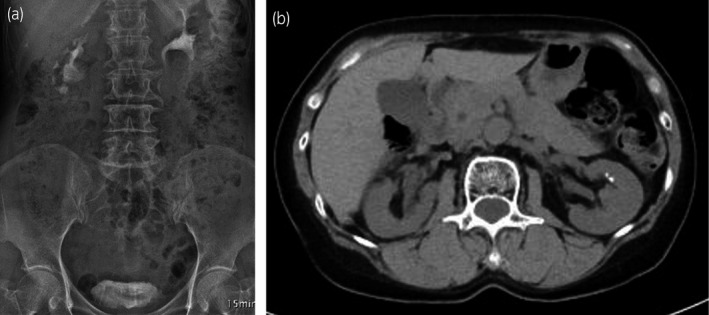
Intravenous urography and CT showing no hydronephrosis at 14 weeks and 34 months after surgery, respectively.
